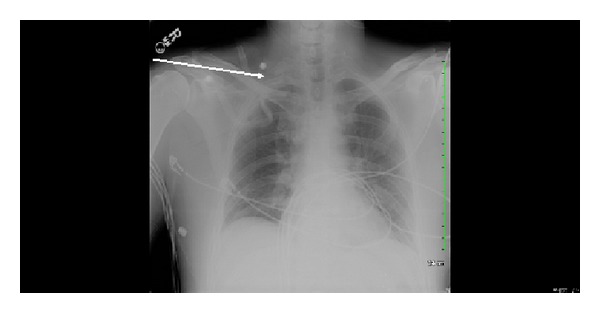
Chest X-ray of our patient after right first rib resection.
